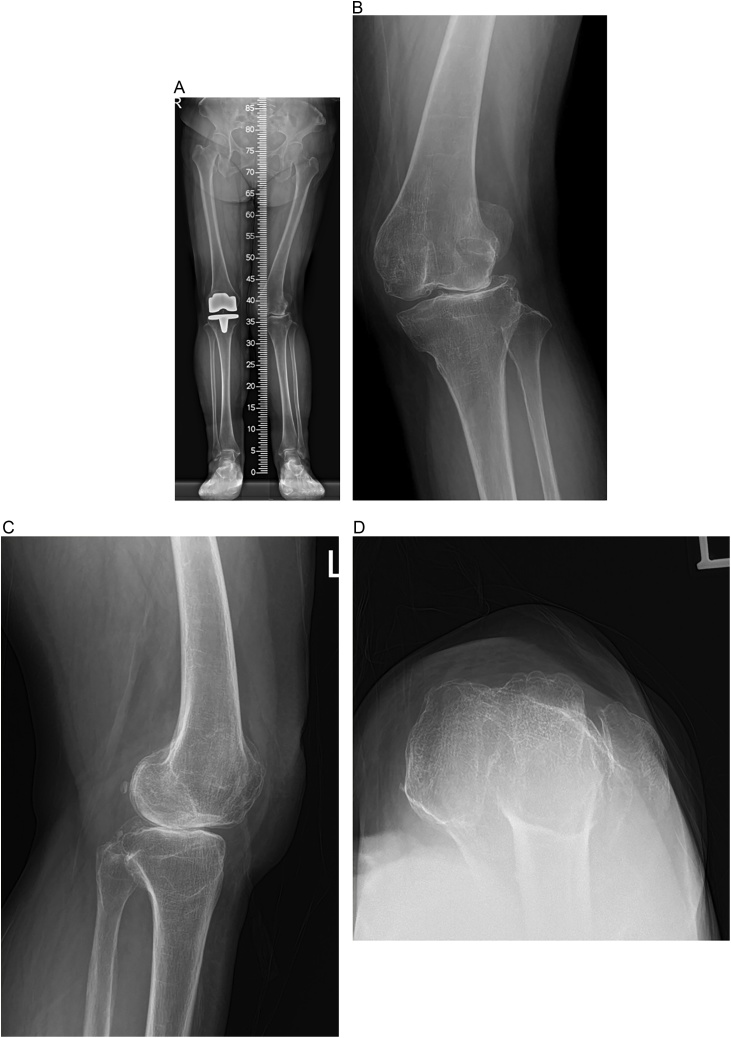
Pre-operative radiograph (A) On low extremity standing scanogram, left knee shows severe valgus alignment. (B) on anteroposterior weight-bearing radiograph, left knee shows severe valgus osteoarthritis with lateral dislocation of the patella (C) on lateral radiography, patella was not seen. (D) On skyline view, patella is dislocated laterally.
